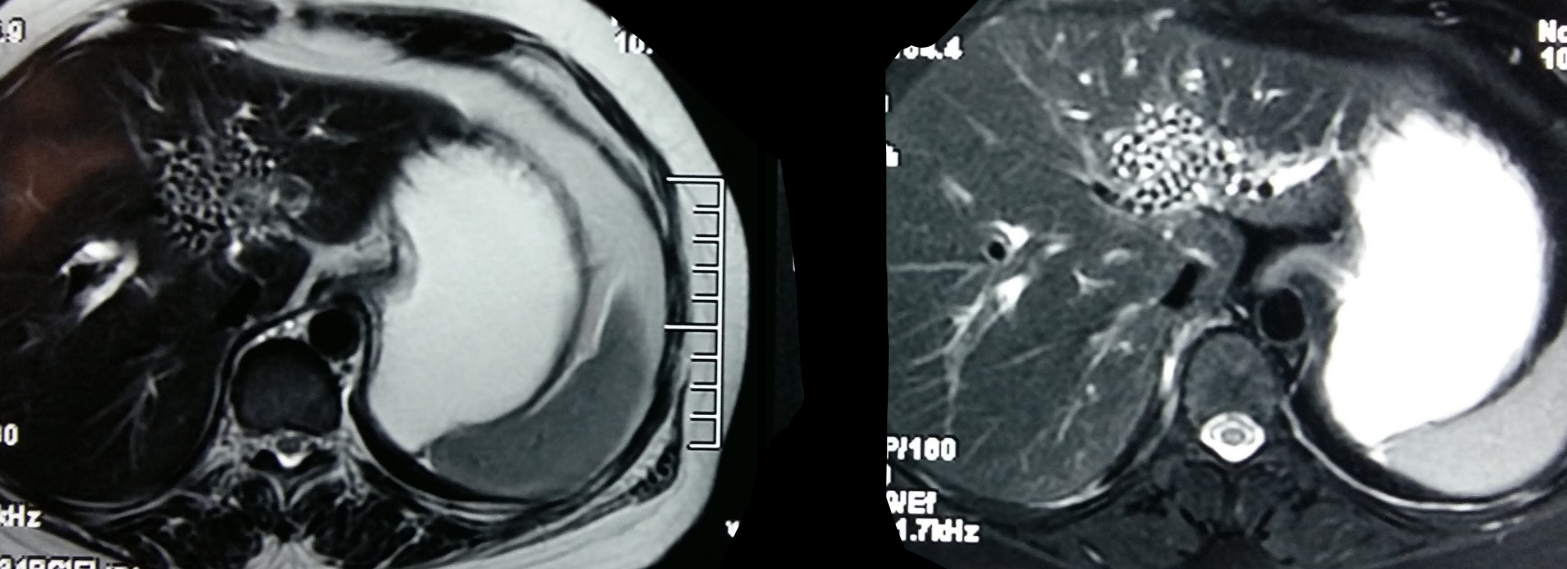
Magnetic resonance imaging (MRI)‐T2 sequence showing hypointense foci of variable sized within the left hepatic duct forming “conglomerate appearance”.
